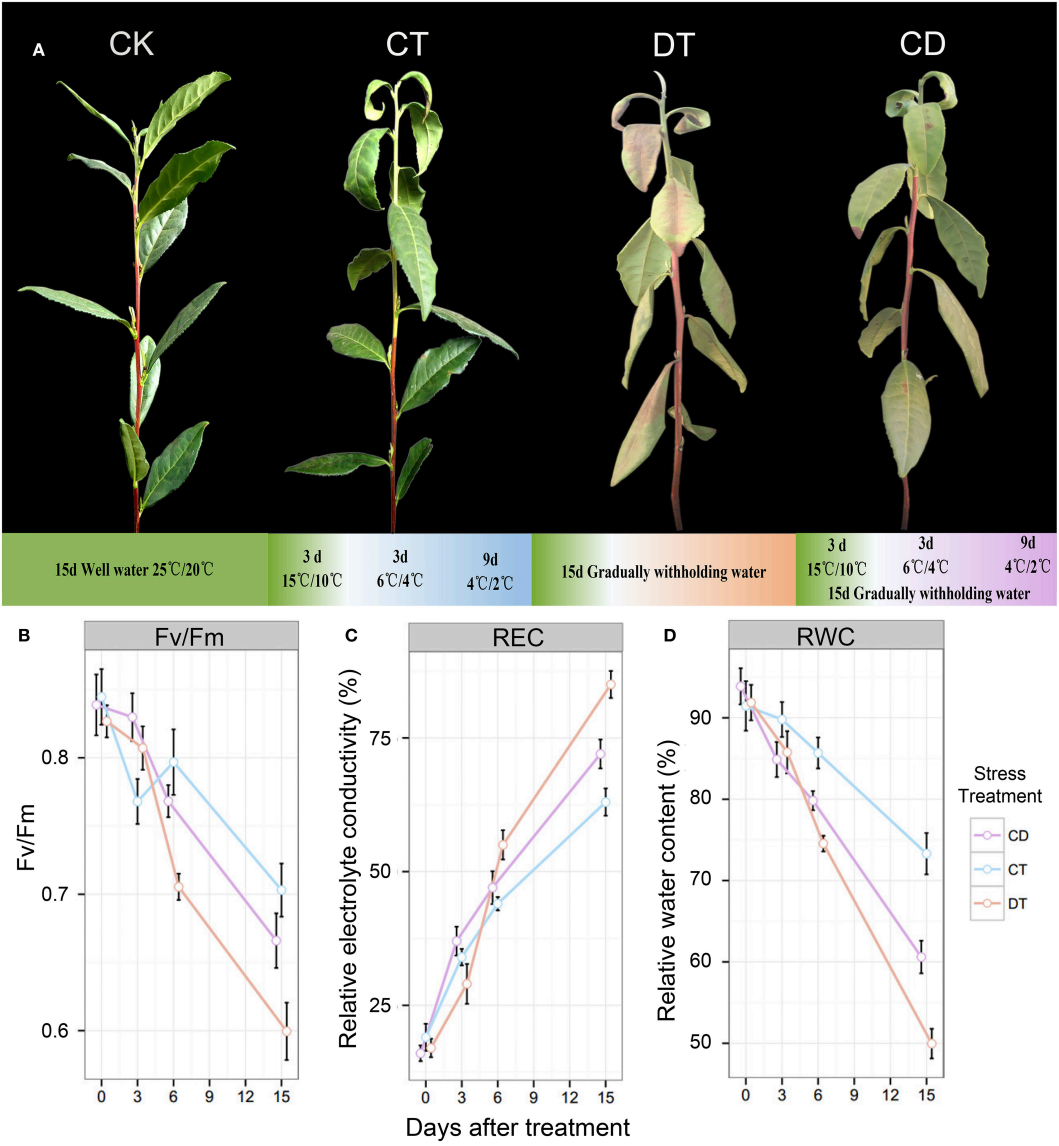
The phenotypes and physiological analyses of tea plants under CT, DT and CD. (A) The phenotypes of control (CK) and plants treated with gradually cold (CT) and drought (DT) stress individually or together (CD) after 15 days. (B) Leaf maximum photochemical quantum yield of PS II (*Fv/Fm*), (C) leaf relative electrolyte conductivity (REC), and (D) relative water content (RWC) were determined at six time-points (0, 3, 6, 9, 12 and 15 days) during CT, DT, and CD.
